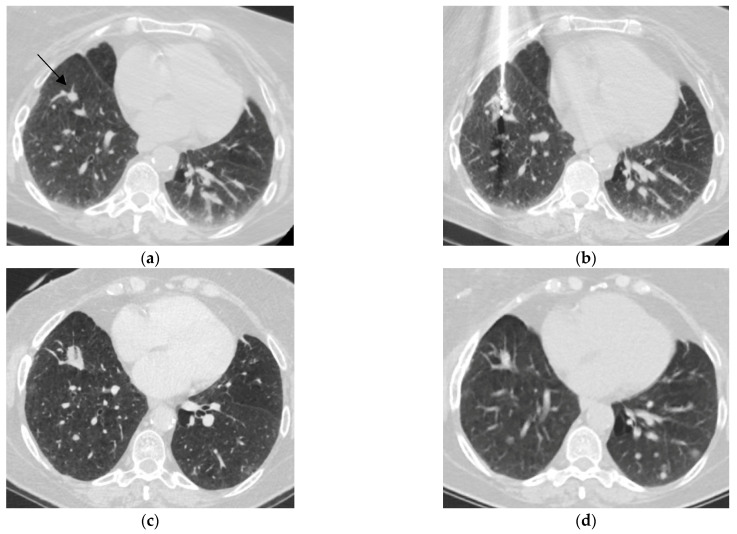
CT-guided percutaneous microwave ablation (MWA) of right lower lobe adenocarcinoma in a previously resected and irradiated patient. A 77-year-old female with stage IB NSCLC status post-two robotic right lower lobe (RLL) wedge resections and mediastinal lymph node dissection presented 20 months later with new pulmonary nodules, confirmed as biopsy-proven invasive adenocarcinoma with endobronchial ultrasound. Given prior thoracic radiotherapy to the right breast and regional lymph nodes two decades ago, stereotactic body radiotherapy (SBRT) was avoided to limit lung function loss, and percutaneous thermal ablation was pursued for treatment. MWA was chosen due to small lesion size and its proximity to vascular structures. (a) Non-contrast CT shows 1 cm RLL lesion (arrow). (b) A 15 G MWA antennae was advanced into the target lesion. Ablation was performed at 65 W for 3 min. (c) A 1-month CT scan shows expected post-ablation changes at the treatment site. (d) A 4-month CT scan shows evolution of the ablation zone with decreased consolidation and no evidence of local recurrence.
